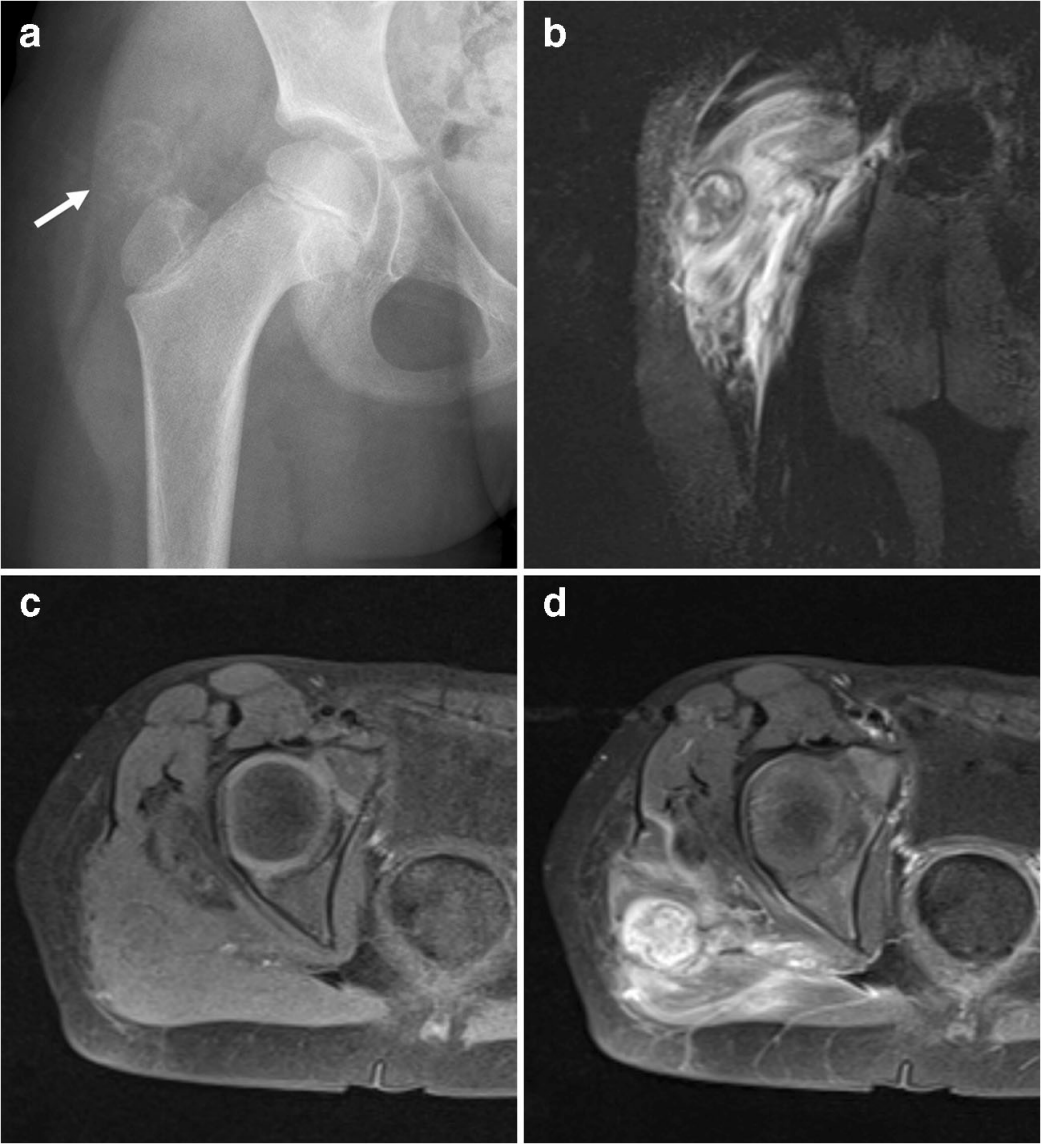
A 6-year-old girl with myositis ossificans, intermediate stage. a Radiography shows an ill-defined calcified lesion cranial to the right greater trochanter. b Coronal turbo inversion recovery magnitude image shows extensive soft tissue edema surrounding the lesion in the right gluteus maximus muscle. c, d Coronal pre- (c) and postgadolinium (d) T1-weighted images with fat suppression show enhancement of the lesion in the right gluteus maximus muscle and surrounding soft tissue. Because of persisting pain, the lesion was finally resected and pathologically proven to be myositis ossificans
